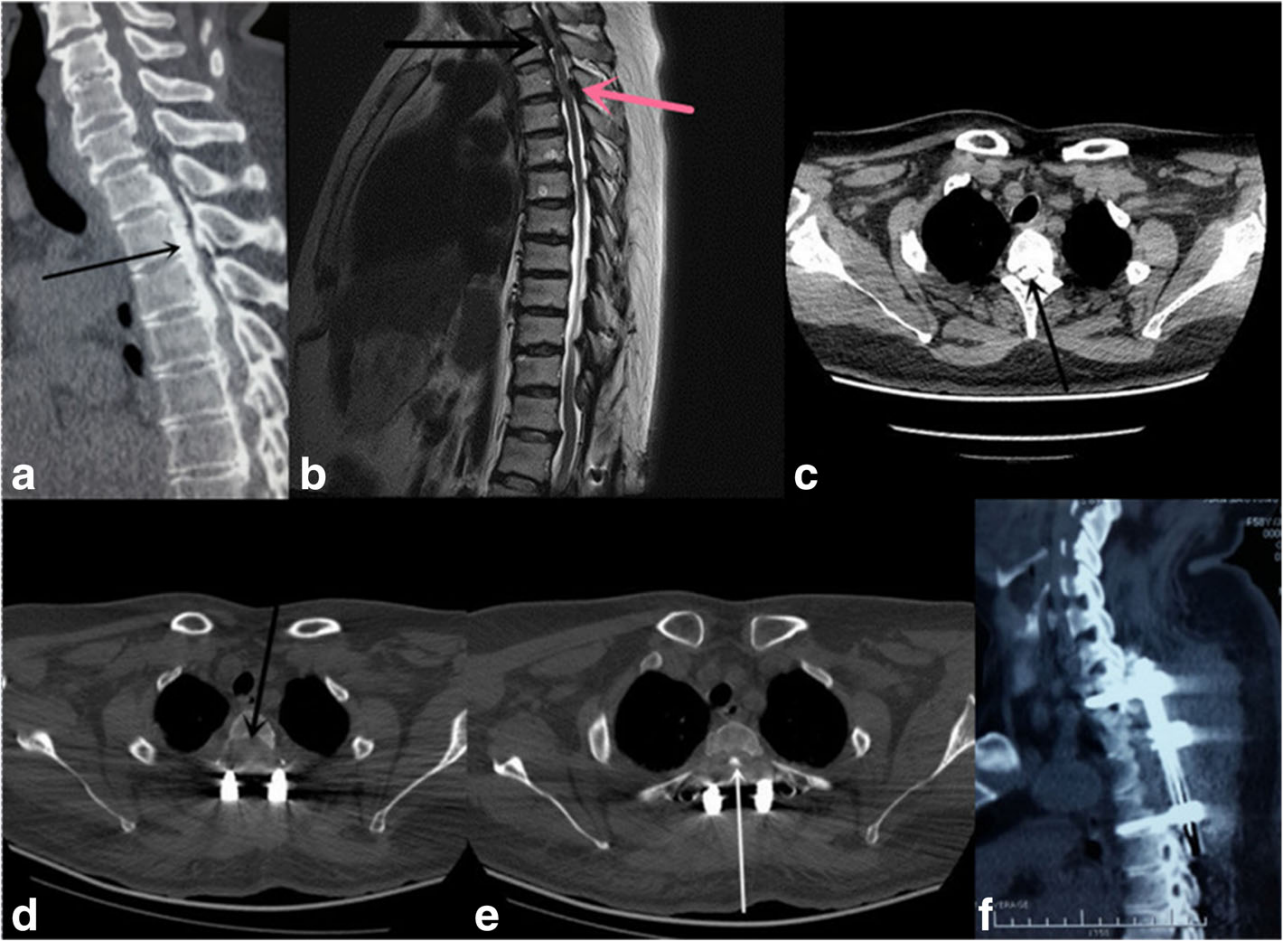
Pre- and post-operative imaging of thoracic OPLL with stenosis in a 65-year-old female patient (patient #18). a Preoperative CT showed OPLL at T1-4 (*arrow, sagittal view*). b Preoperative MRI showed spinal cord compression at segments T1-4 and yellow ligament hypertrophy at T3 (*arrow, sagittal view*). c Preoperative CT image showed ossification of PLL at the T2 level (*arrow, cross-sectional view*). d Postoperative CT image showed complete removal of the PLL at the T2 level (*arrow, cross-sectional view*). e Postoperative cross-sectional CT image showed a suspending PLL at the T3 level (*arrow, cross-sectional view*). f Postoperative CT image showed removal of 1/3 of the T1-4 vertebral bone and PLL (*sagittal view*)
